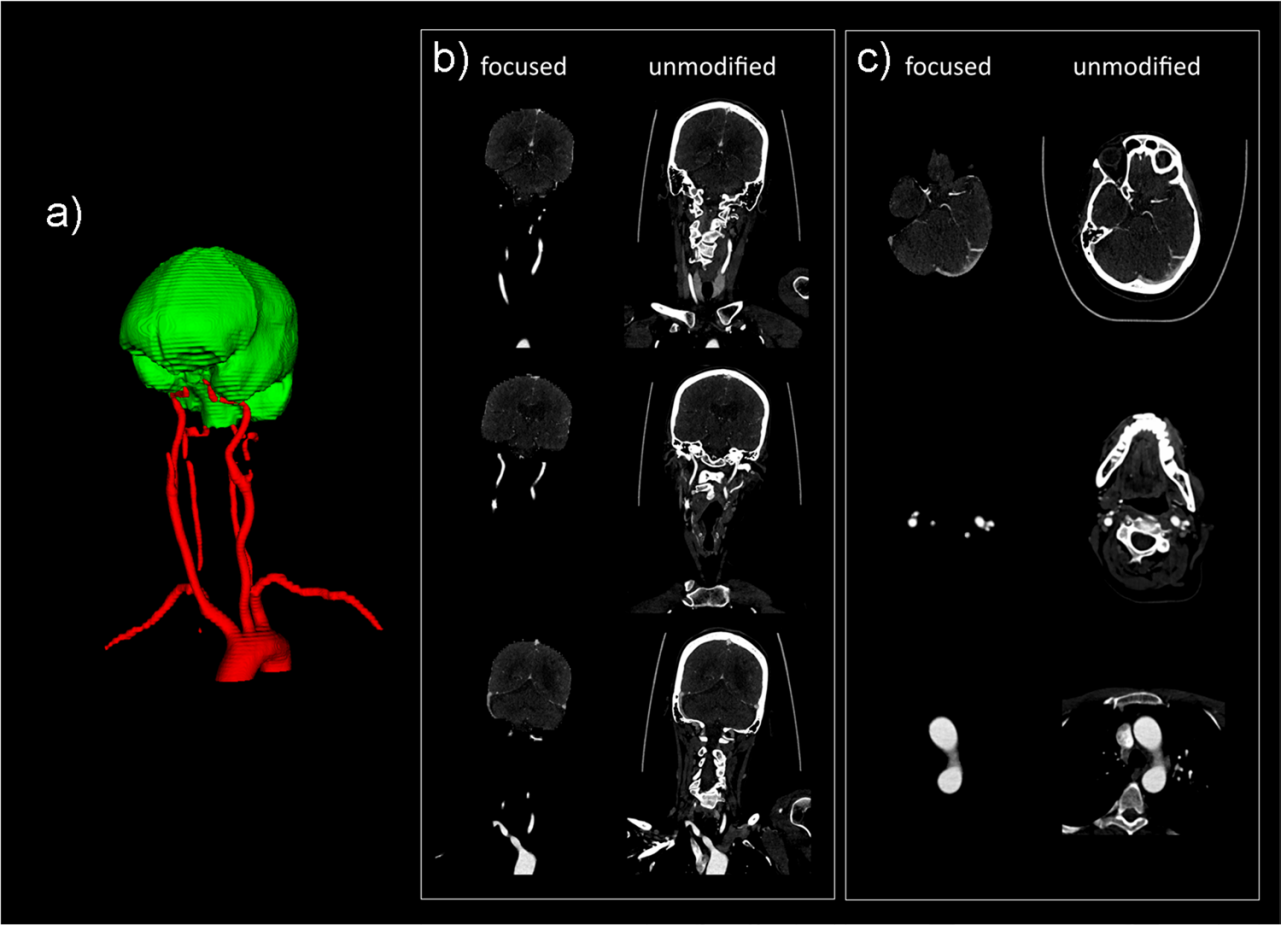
Second example of focused view CTA in the test set. Three-dimensional segmentation (a), coronal focused view CTA slices next to unmodified CTA slices (b), and axial focused view CTA slices next to unmodified CTA slices (c) are shown. The full focused view CTA dataset is shown in supplementary (video) file 2
